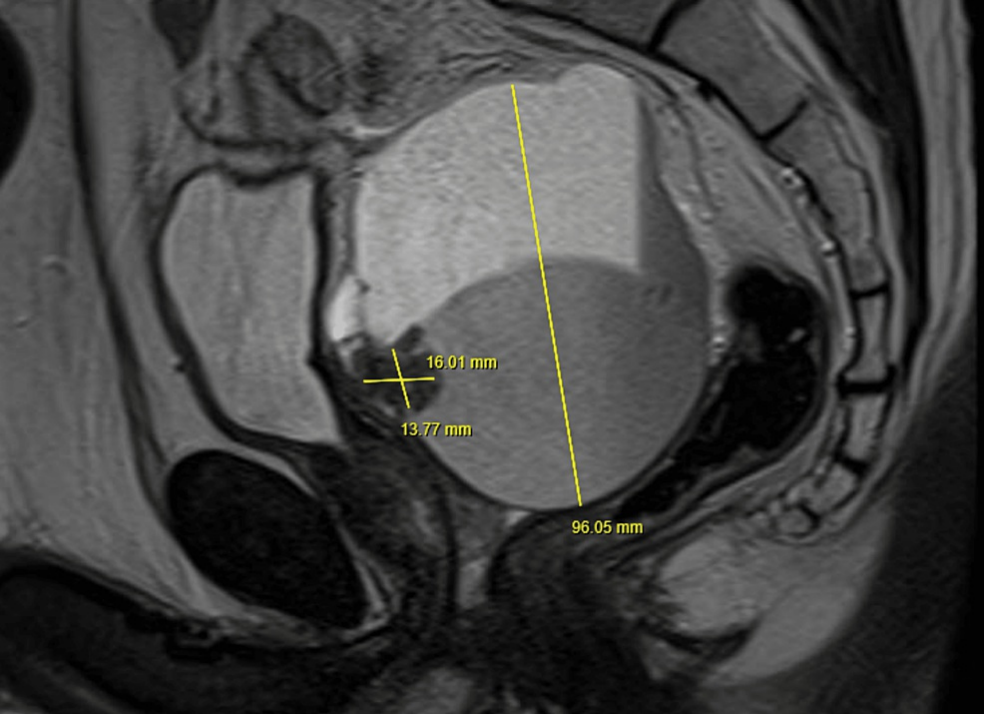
MRI pelvis-prostate Dilated haemorrhagic left seminal vesicle measuring 9.6cm with a hypercellular lesion (13.8 x 16mm) within, located superior to the prostatic base
